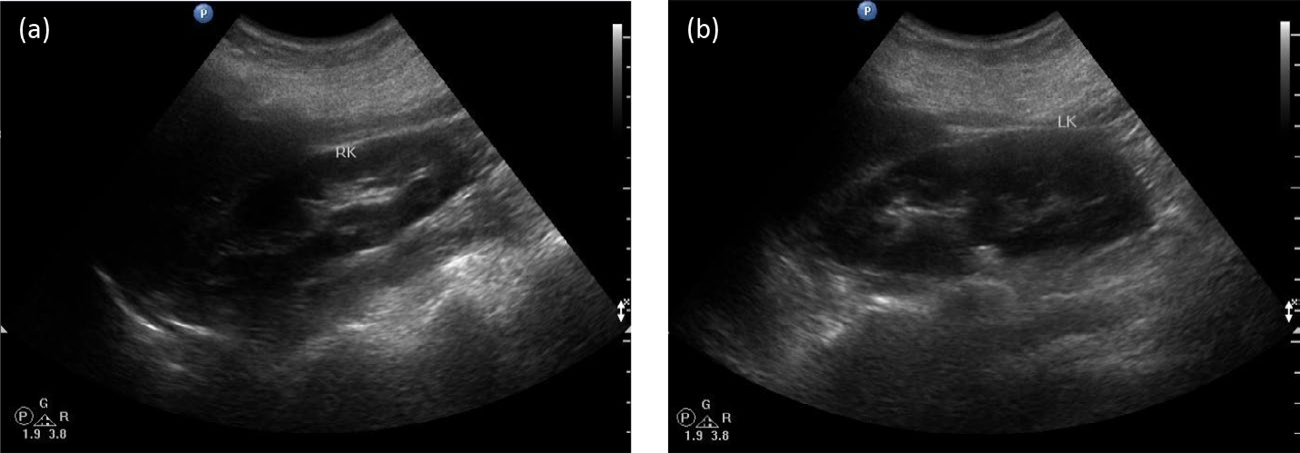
Transabdominal ultrasound image of the POI subject. Ultrasound of the right kidney (a) and left kidney (b) from the proband showed normal size, structure and position of kidneys and ureters
